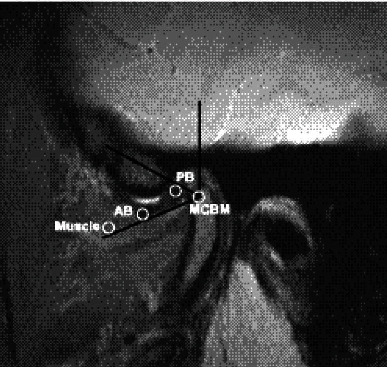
Selection of ROIs on MR images. AB: anterior band; PB: posterior band; MCBM: mandibular condyle bone marrow.
